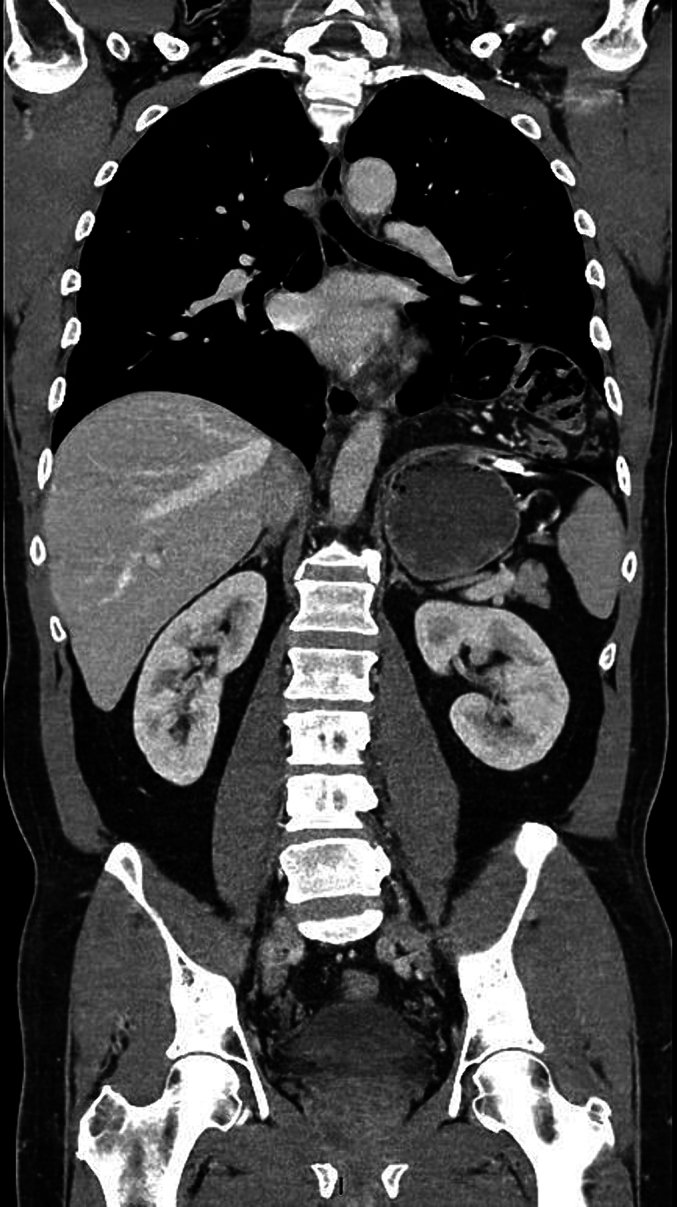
CT-thor/abd. with IV contrast, coronal cross-section
